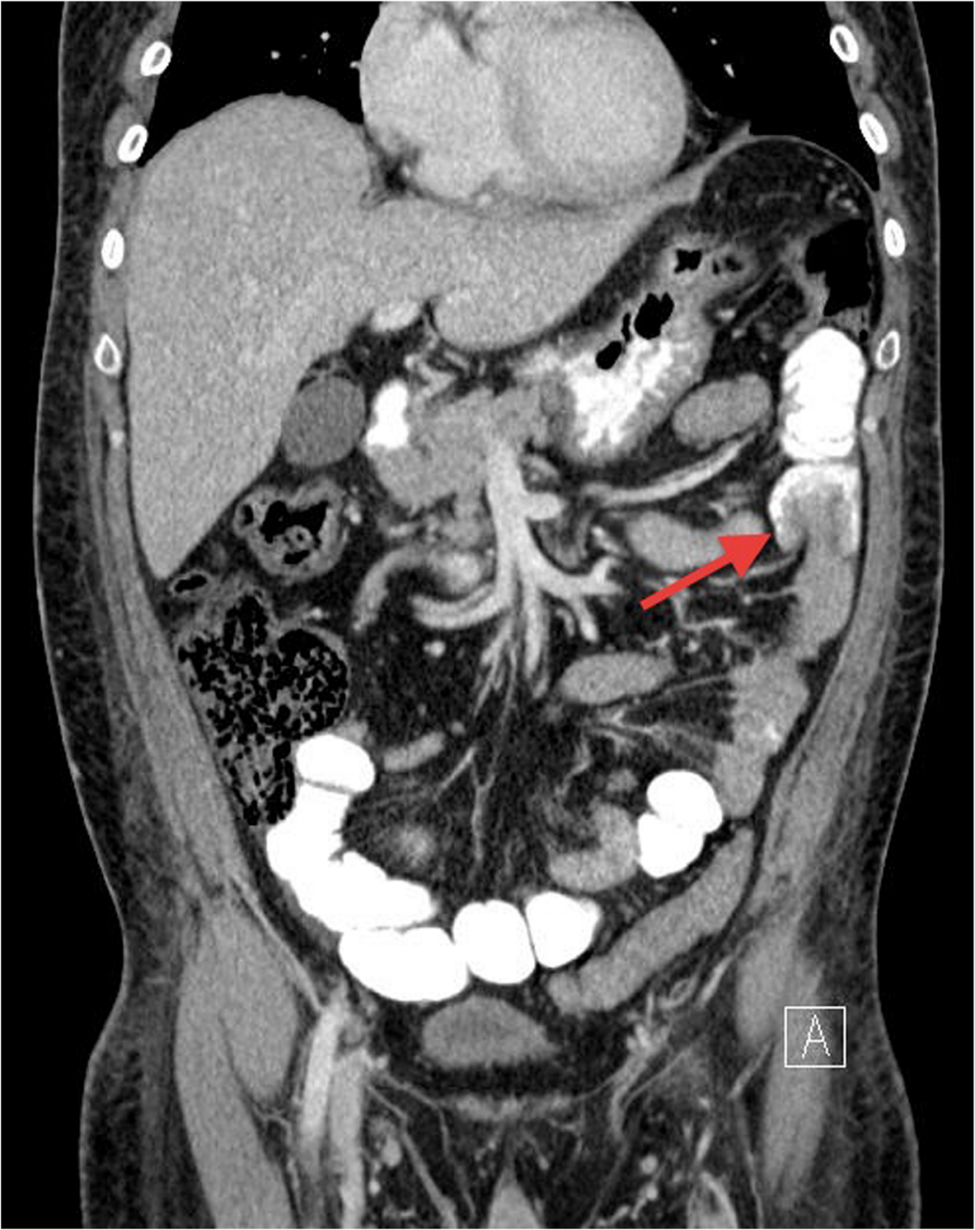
Abdominal CT scan (coronal section); showing a segment of small bowel loop. Invaginated into a proximal bowel loop, indicating small bowel intussusception (arrow). There were no signs of bowel ischemia nor obstruction.
